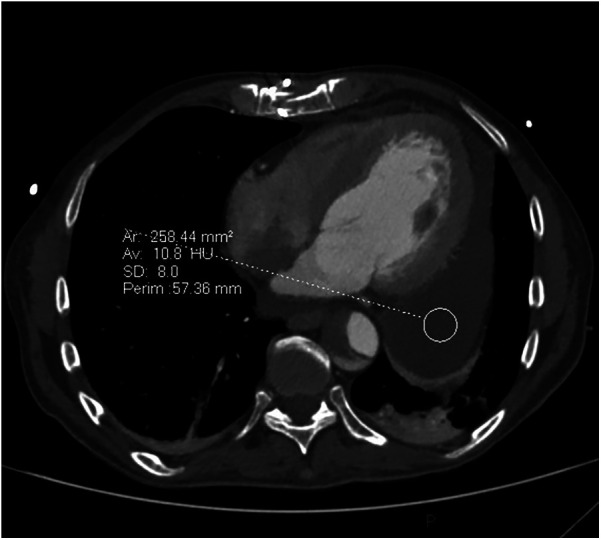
Cross section cardiac CT image before pericardocentesis demonstrating circumferential low attenuating pericardial effusion, thickest behind lateral wall of the left ventricle.
